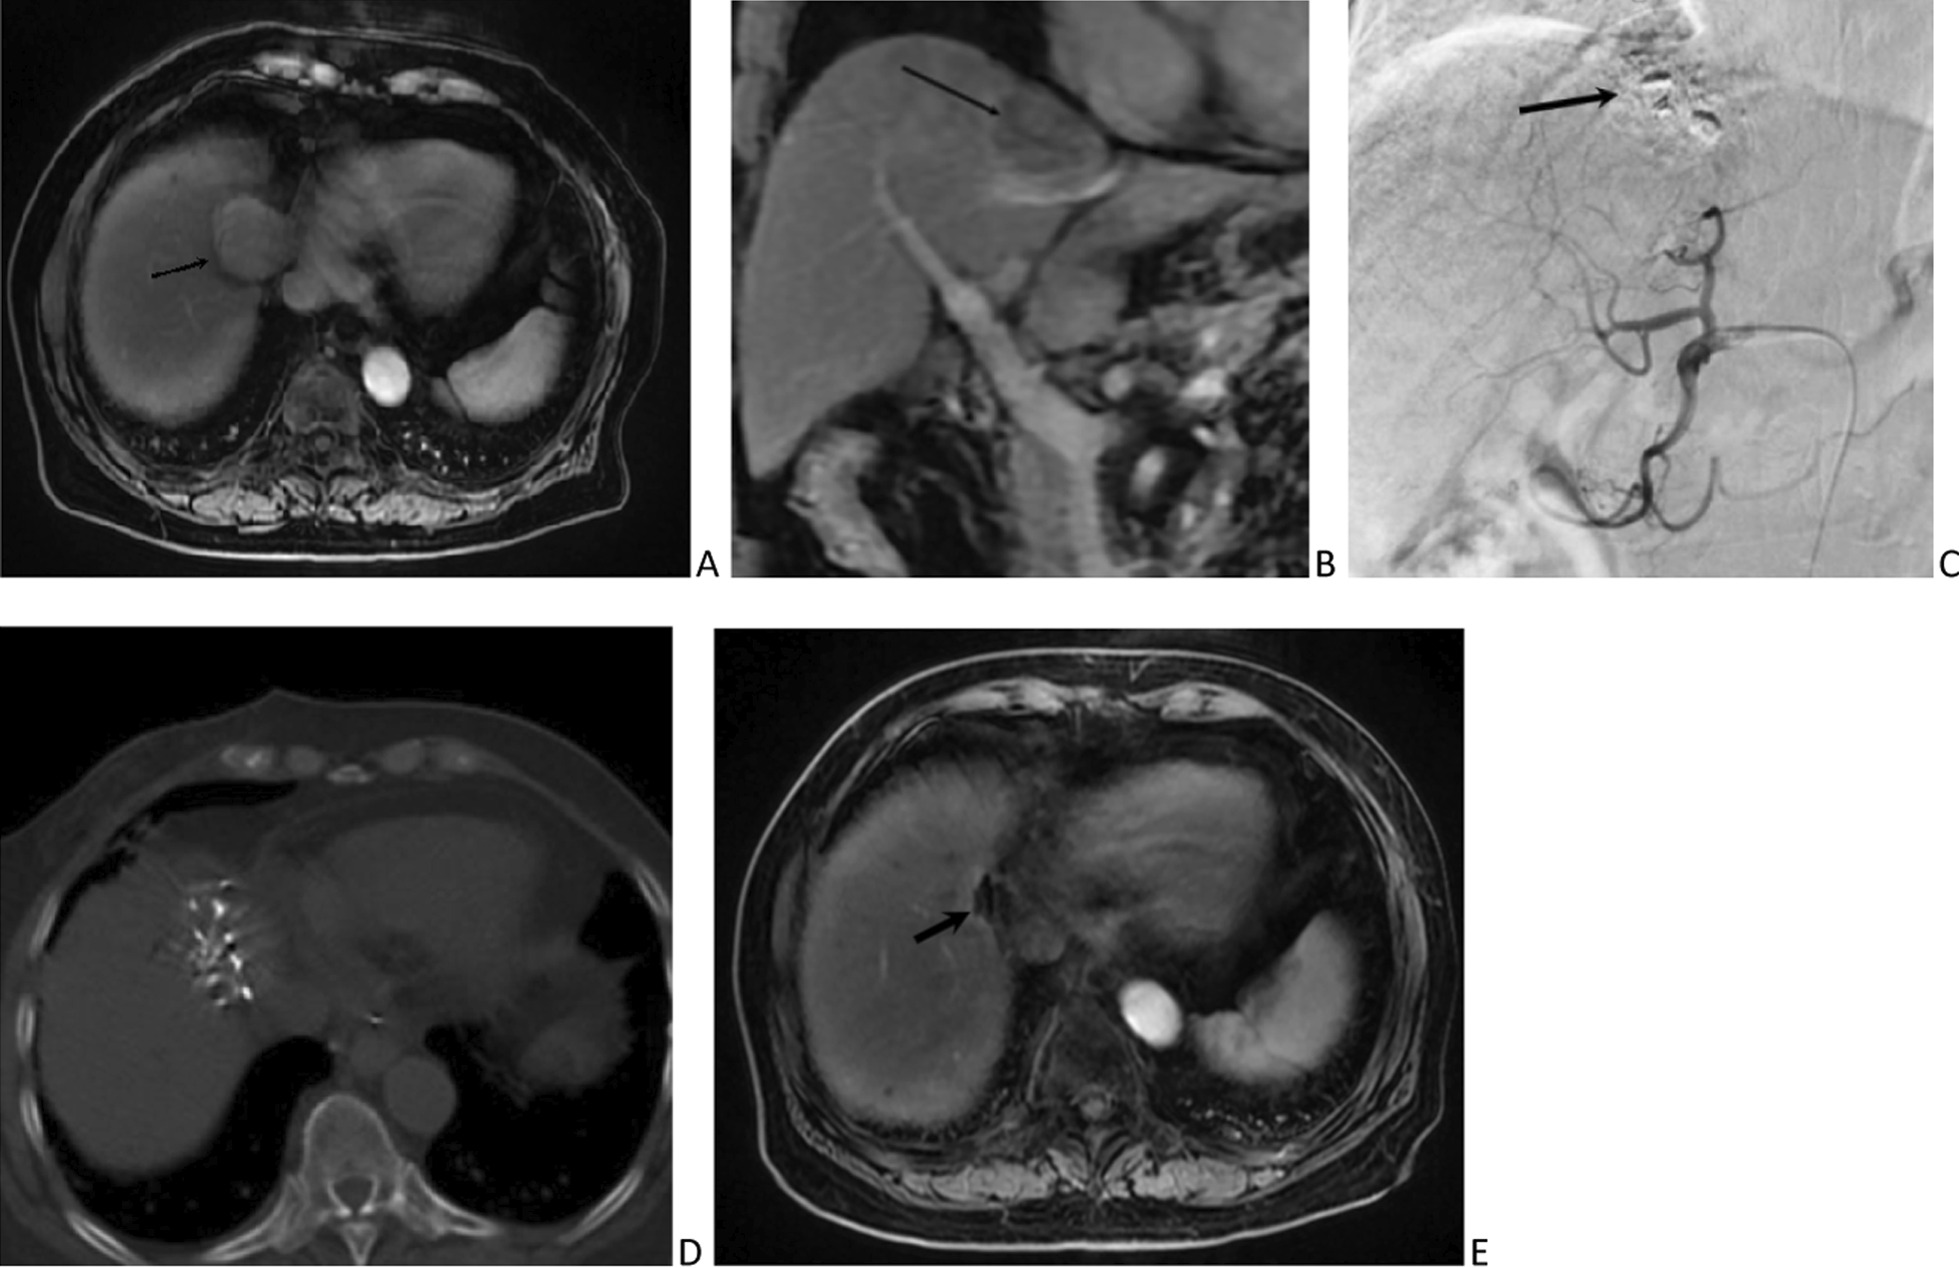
A 78-year-old female with subcapsular HCC underwent combined TACE and ISB treatment. Preoperative a axial and b coronal contrast-enhanced MRI showed the subcapsular HCC (arrows). c The procedure of TACE (arrow). d The ISB was performed by the CT guidance. e Postoperative contrast-enhanced MRI showed the shrinkage of the tumor with no intratumoral arterial enhancement (arrow). It could be considered as CR
